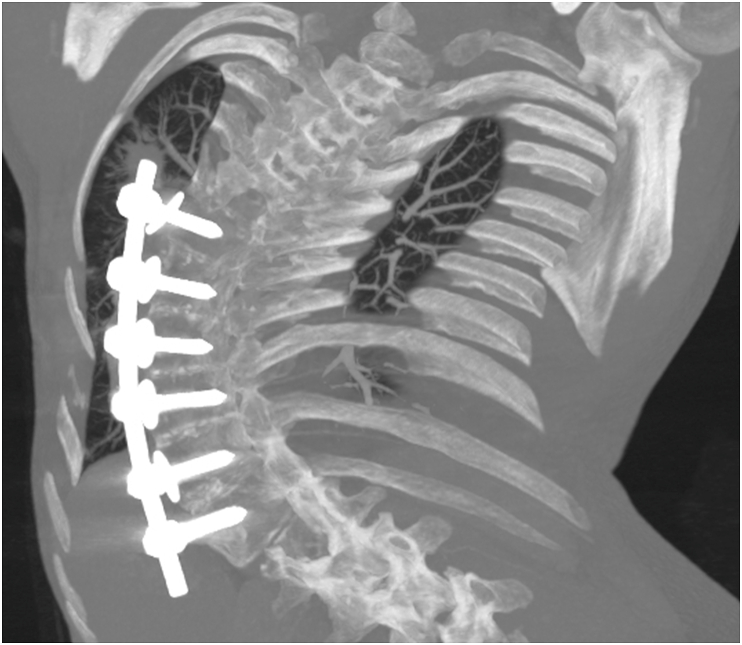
Oblique coronal MIP CT image shows displaced anterolateral spinal rod and adjacent screw to the right upper lobe; the caudal edge of the rod penetrated to the liver.
